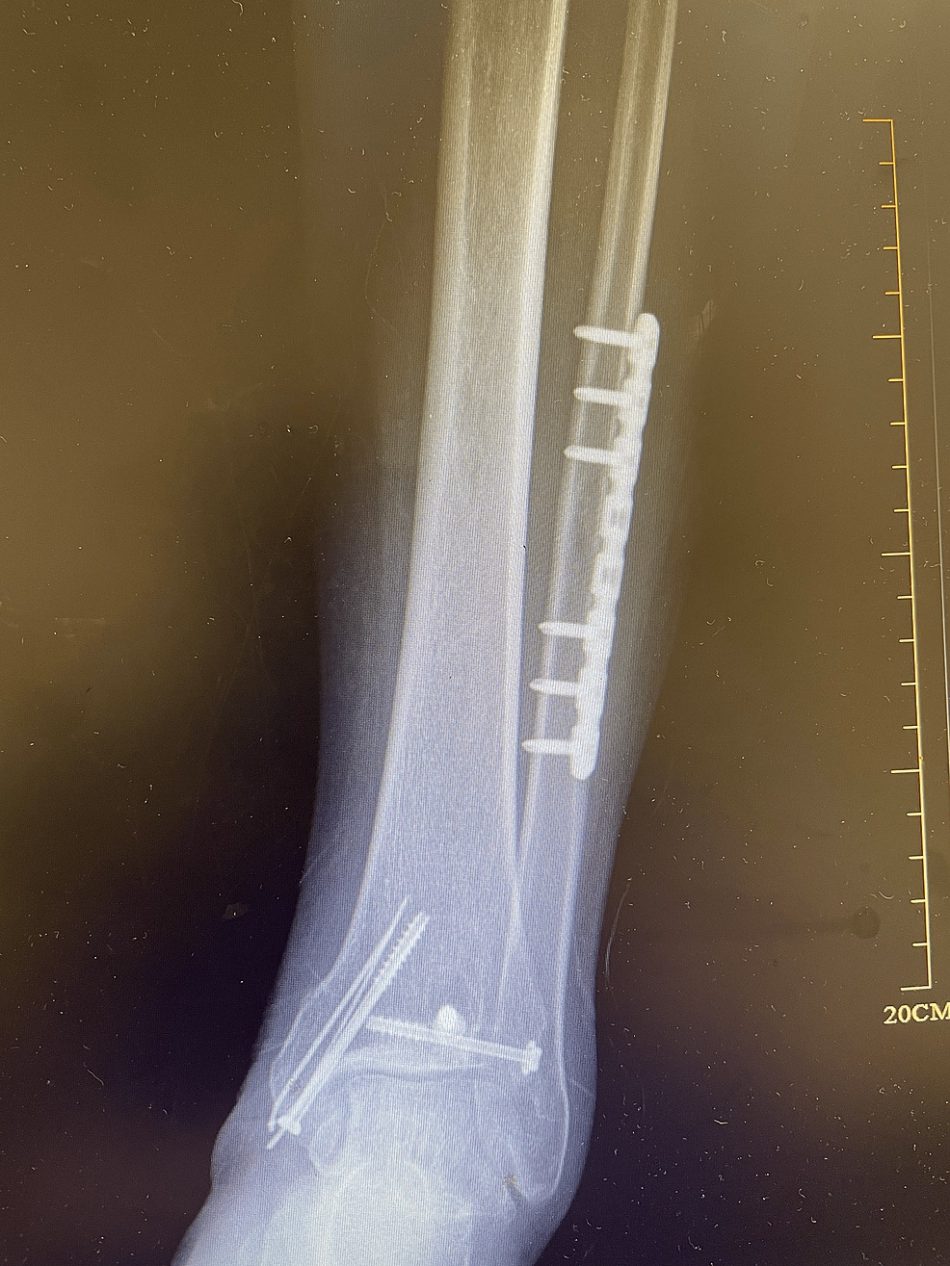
Postoperative anteroposterior radiograph. The lateral malleolus was fixed with reconstruction plates, and the posterior malleolus was fixed with a cannulated screw. Because the medial malleolar fragment is too small, it is fixed with a cannulated screw and three Kirschner wires.
